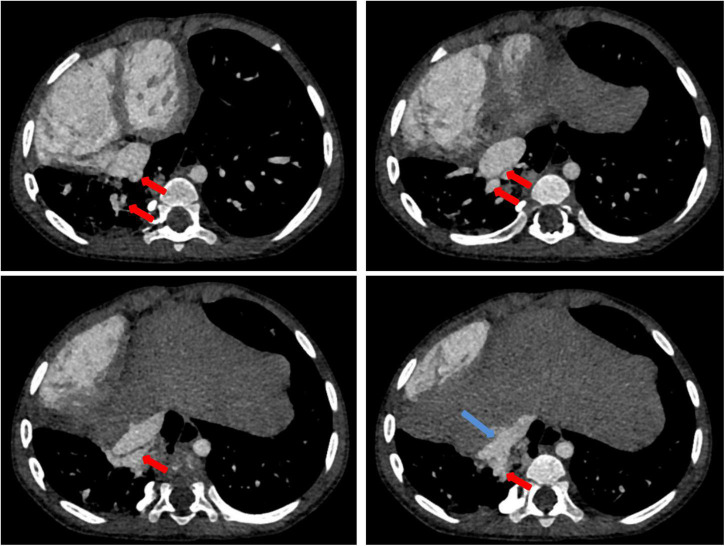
Contrast-enhanced CT of the chest: contrast-enhanced CT shows the isolated pulmonary venous draining (red arrow) into the hepatic vein (blue arrow).
